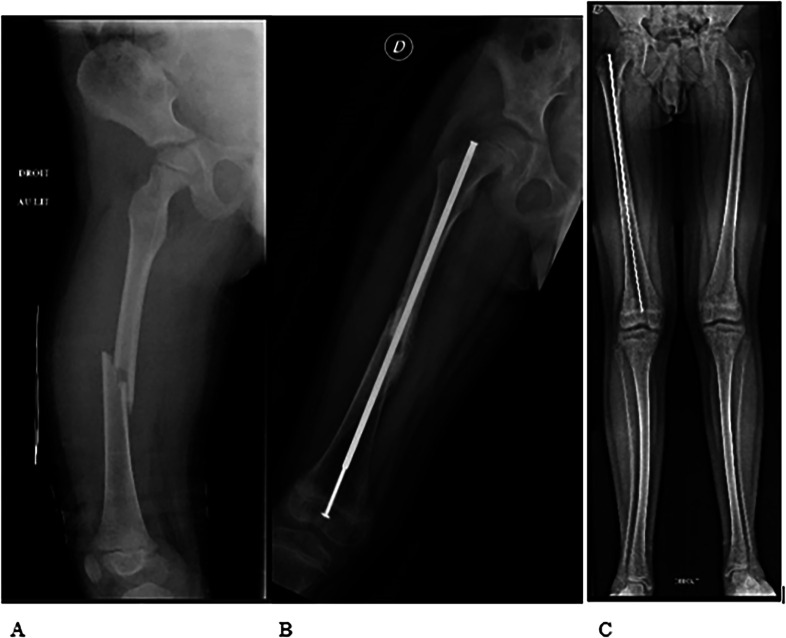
Pre-operative (A), post-operative (B) and 6-year-follow-up (C). X-rays of one of this study population patients showing no growth arrest or aseptic femoral head necrosis along as good nail positioning and femur alignment.
